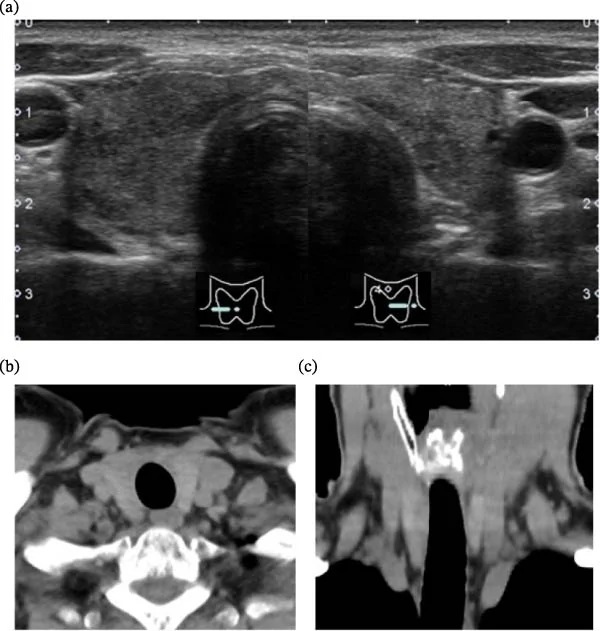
High‐resolution ultrasound of the thyroid (a), transverse view. The right lobe is slightly enlarged with a heterogenous echotexture, hypervascularity, and no nodules. The left lobe showed no enlargement, with no cysts or increased blood flow. Computed tomography scan of the thyroid, transverse (b) and coronal (c) views. The right lobe demonstrates mild enlargement.
